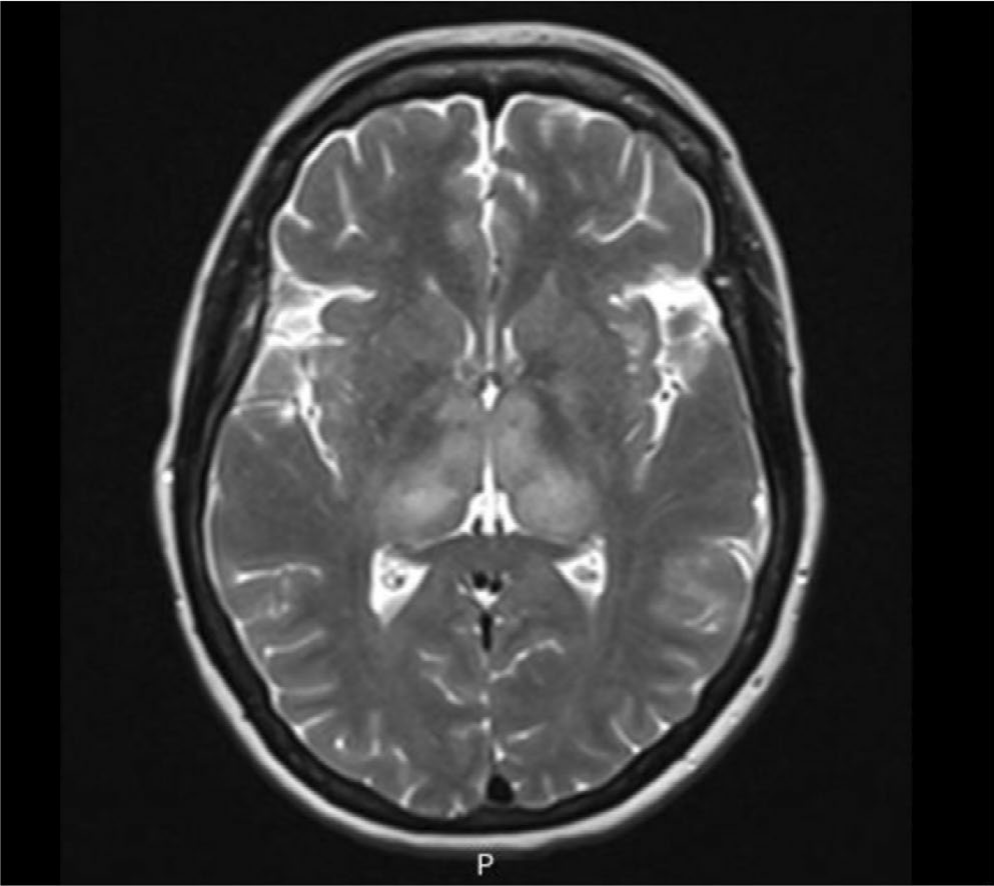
FIGURE 1 Brain lesions in MRI T2 sequence
